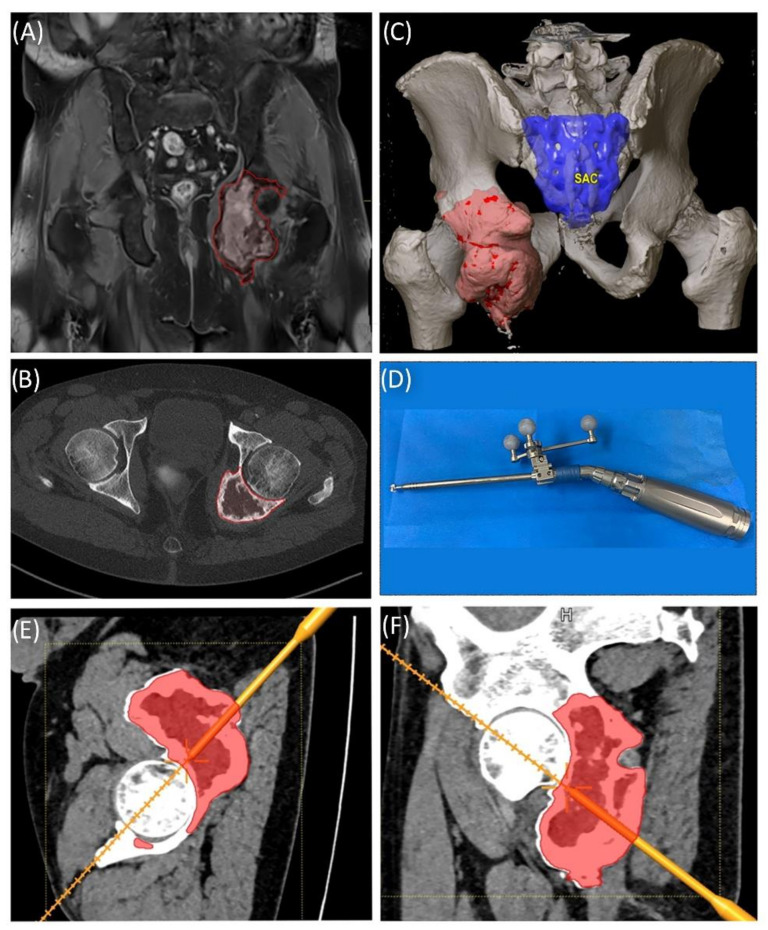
Case 5: Segmentation of the giant cell tumor in magnetic resonance imagining (MRI) (A) and computed tomography (CT) (B) scans, segmented tumor volume (C), high-speed burr navigation (D), intraoperative navigation of the tumor volume with the high-speed burr tool tip (E,F).
